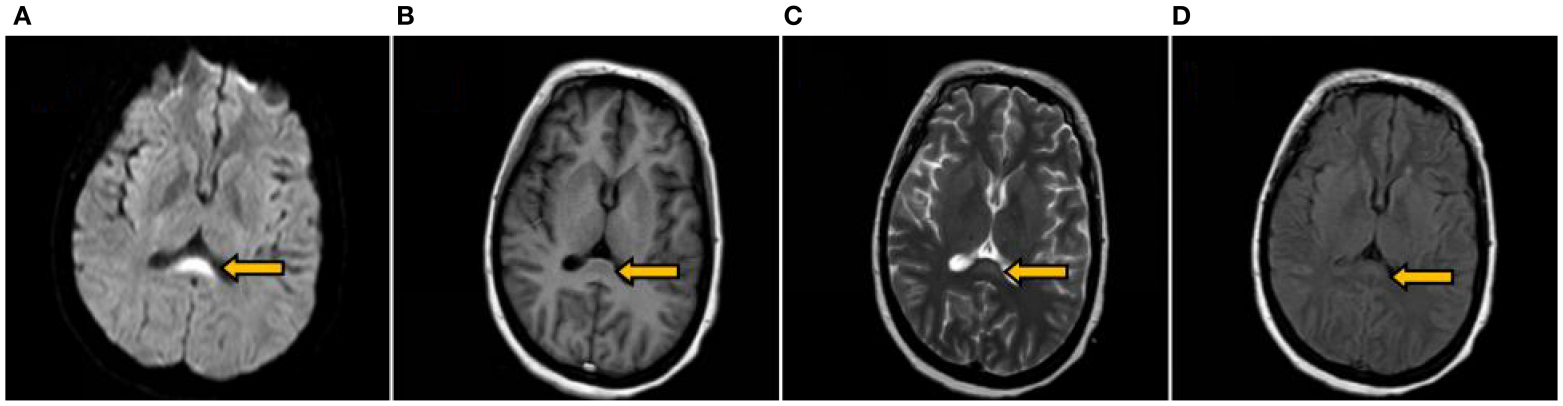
Brain MRI on the 9th day after symptom onset. Revealing an oval symmetrical lesion (indicated by the arrow) located in the central region of the splenium of the corpus callosum. The lesion appeared hyperintense on DWI (A) hypointense on T1WI (B) and exhibited slight hyperintensity on both T2WI (C) and T2Flair (D).
